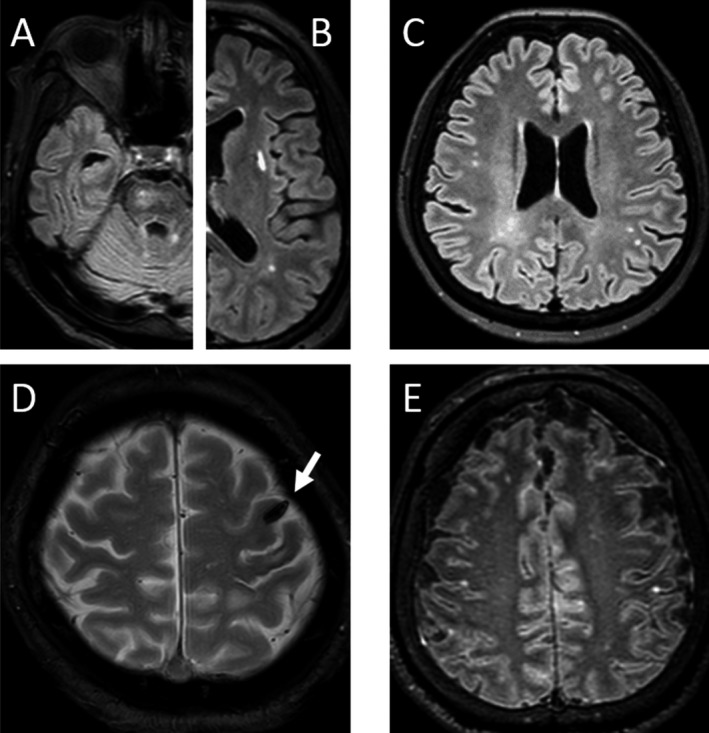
Representative magnetic resonance imaging (MRI) findings of lesion locations such as brain stem (A), basal ganglia (B), white matter without site predilection (C), and non‐parenchymal lesions (D, E). Brain stem, basal ganglia, and white matter lesions were assessed with fluid‐attenuated inversion recovery (FLAIR) images (A–C). Cerebral vein thrombosis was detected as hypo‐intensity on T2 star‐weighted image (D; arrow). Post‐contrast three‐dimensional FLAIR image revealed leptomeningeal enhancement compatible with acute meningeal syndrome (E).
